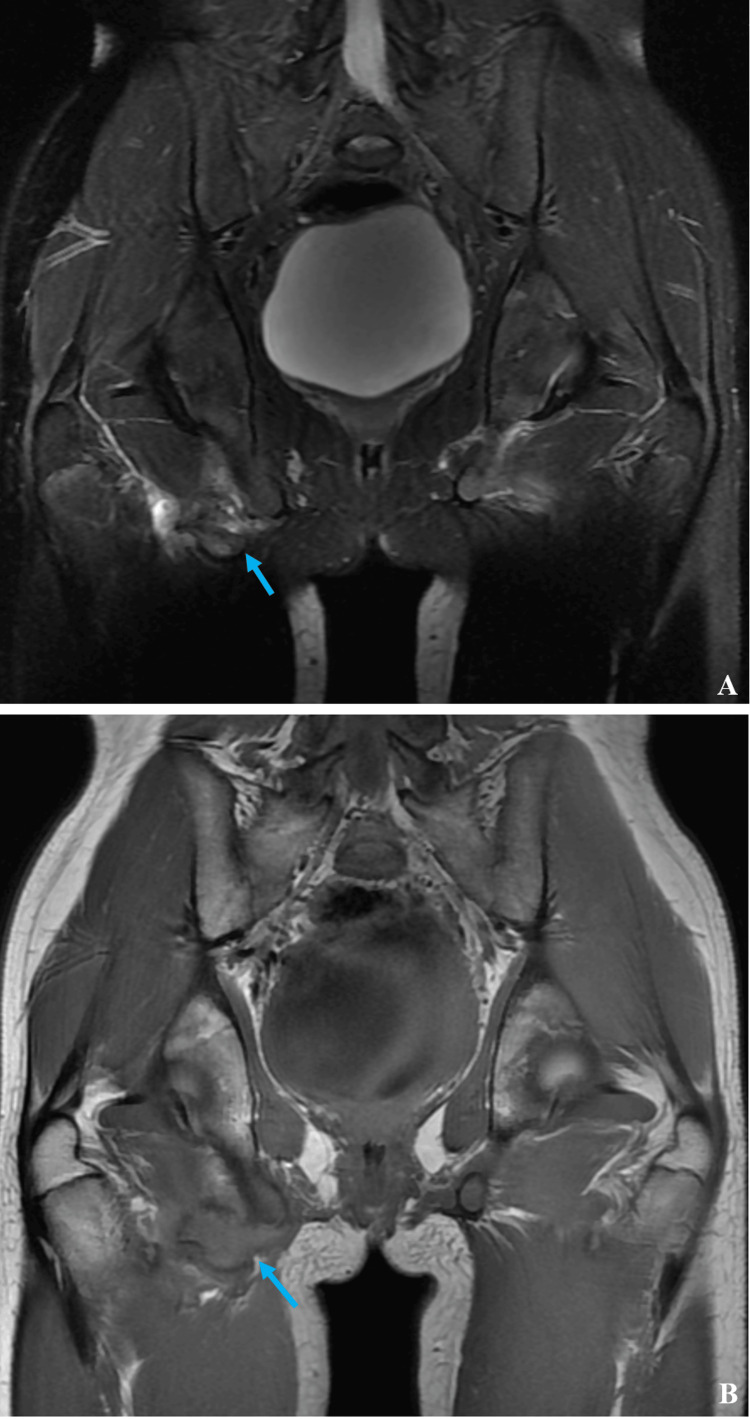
Magnetic resonance images of patient hips Coronal T2-weighted image with fat saturation (A) and coronal T1-weighted image (B) demonstrate that the cluster of ovoid calcifications adjacent to the avulsed right ischial tuberosity fragment corresponds to several foci of callous formation in the soft tissues without a true mass (blue arrows).
